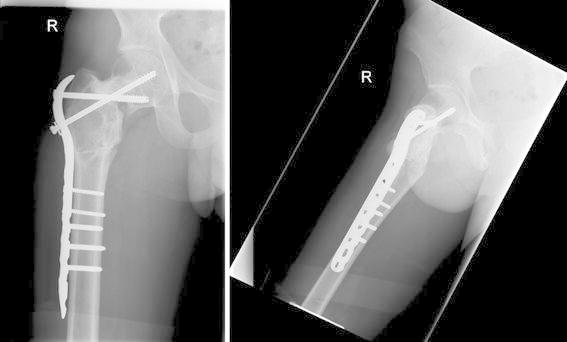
Antero-posterior and lateral radiographs of the right hip showing correction of the deformity and internal fixation of the femoral neck fracture, 12 months after the injury
